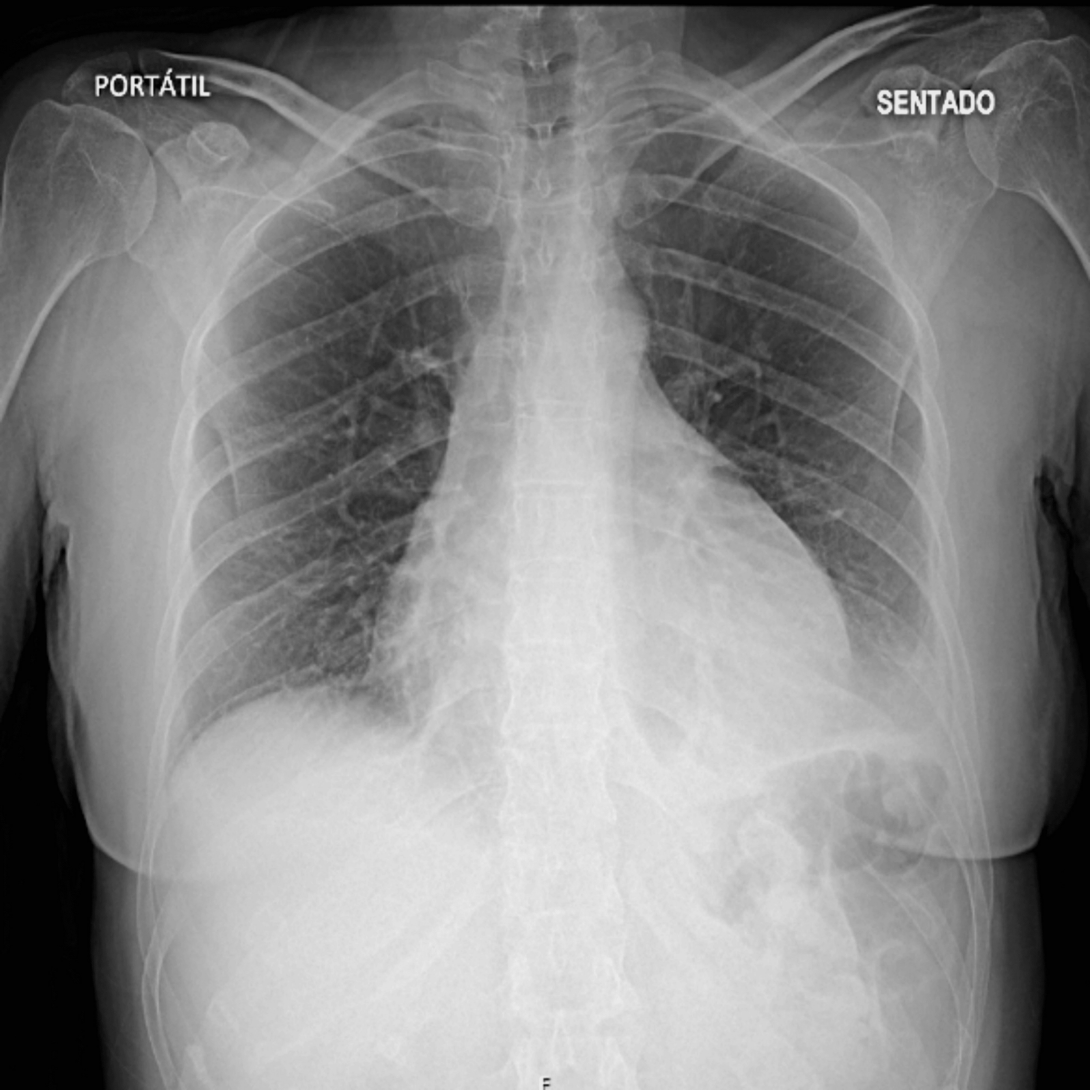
Chest X-ray showing an increased cardiothoracic ratio
